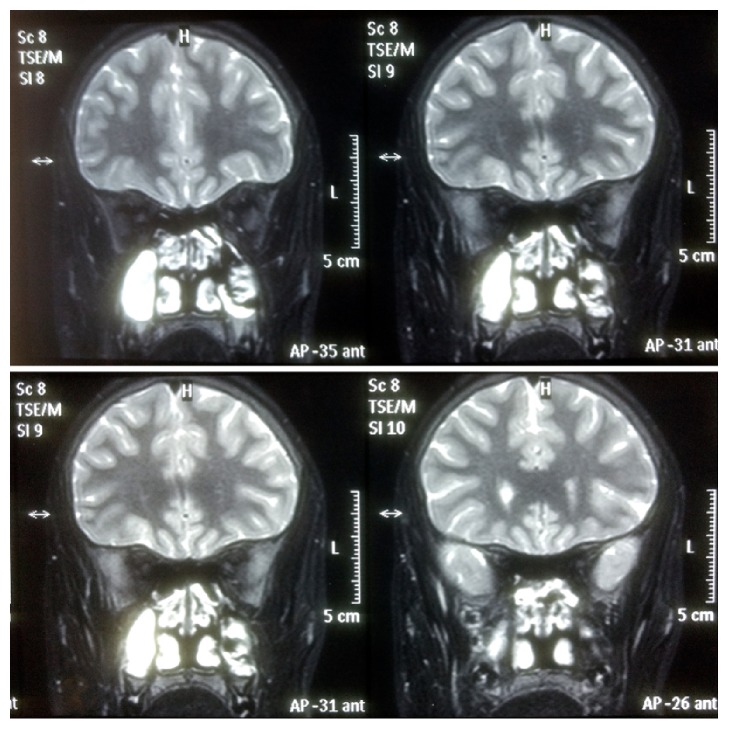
MRI head and orbit coronal section at the level of the midorbit and apex showing absence of any granulomatous lesions nor any extraocular muscle thickening (ruling out myositis or orbital pseudotumor).
